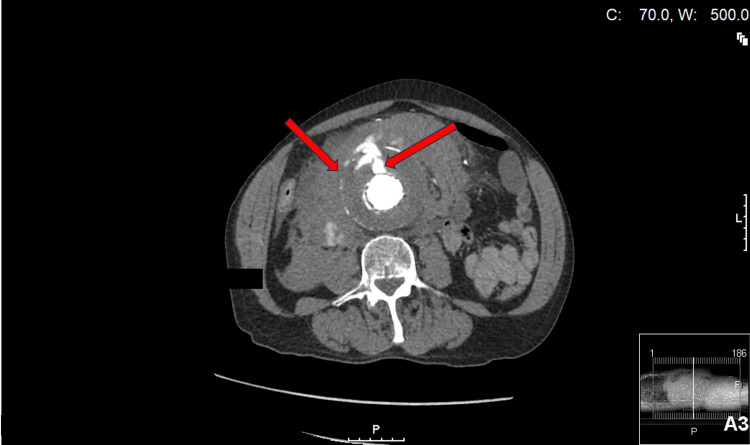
CT abdomen transverse view of AAA showing intraperitoneal blood products (left arrow) and anterior endoleak (right arrow) CT: computed tomography; AAA: abdominal aortic aneurysm
